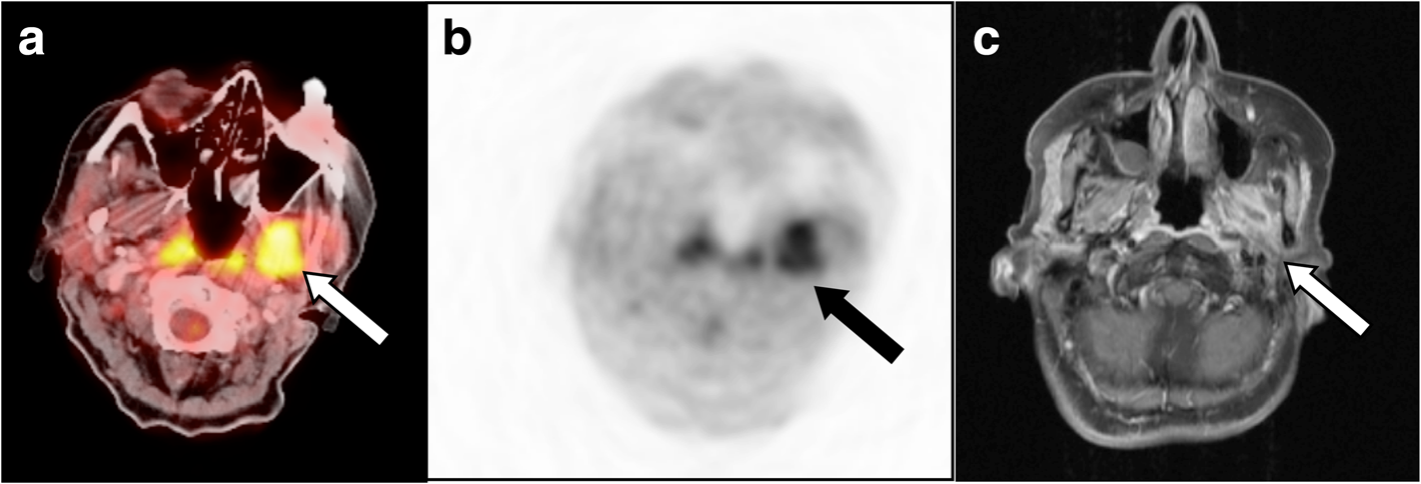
ACC recurrence: PET/CT imaging was true-positive, indistinctive finding on MRI. Patient after resection of an adenoid cystic carcinoma in the left parotid gland and after combined neutron/proton therapy. Recurrence is seen on the fused PET/CT (a) and PET image (b) with a focally increased FDG-uptake (arrow), but not on the contrast-enhanced T1-weighted MR image (c). In MRI, the finding was evaluated as radiation necrosis
